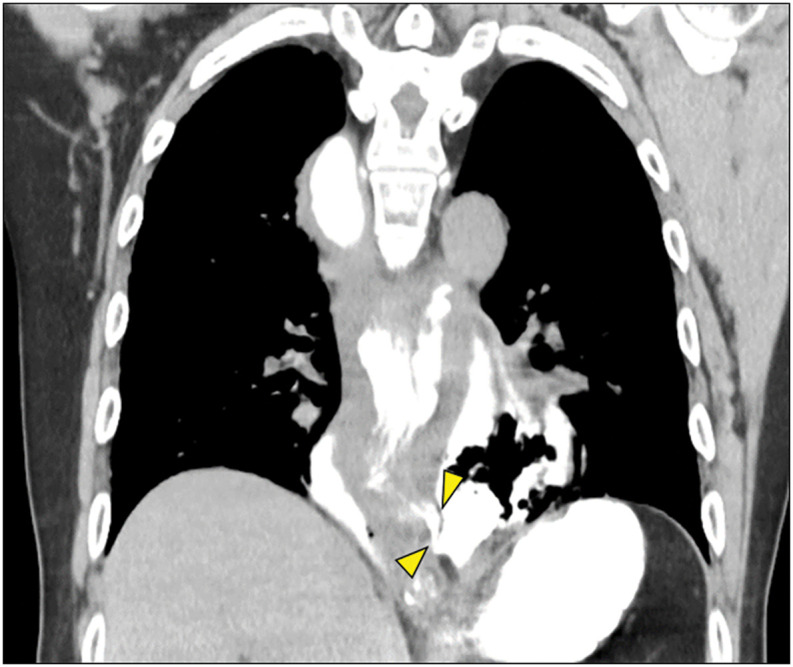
CT imaging. The yellow arrow indicates the perforation site.
